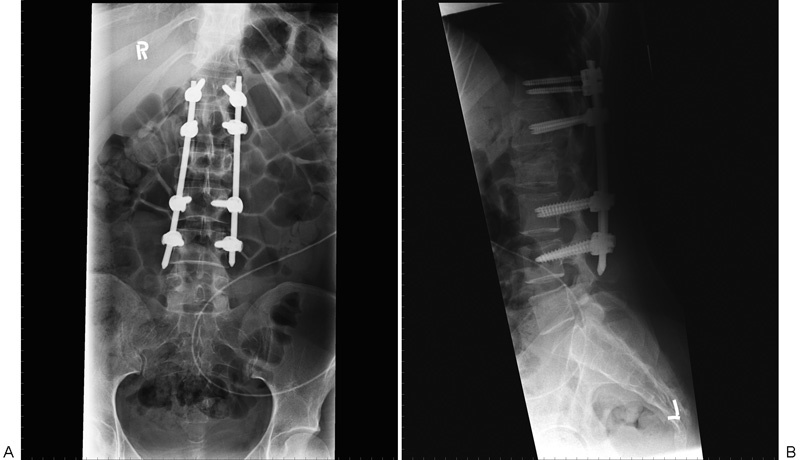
Plain film at 9-month follow-up of T12–L2 construct. Preservation of deformity correction and no migration of pedicle screws are demonstrated. (A) Anteroposterior view. (B) Lateral view.
